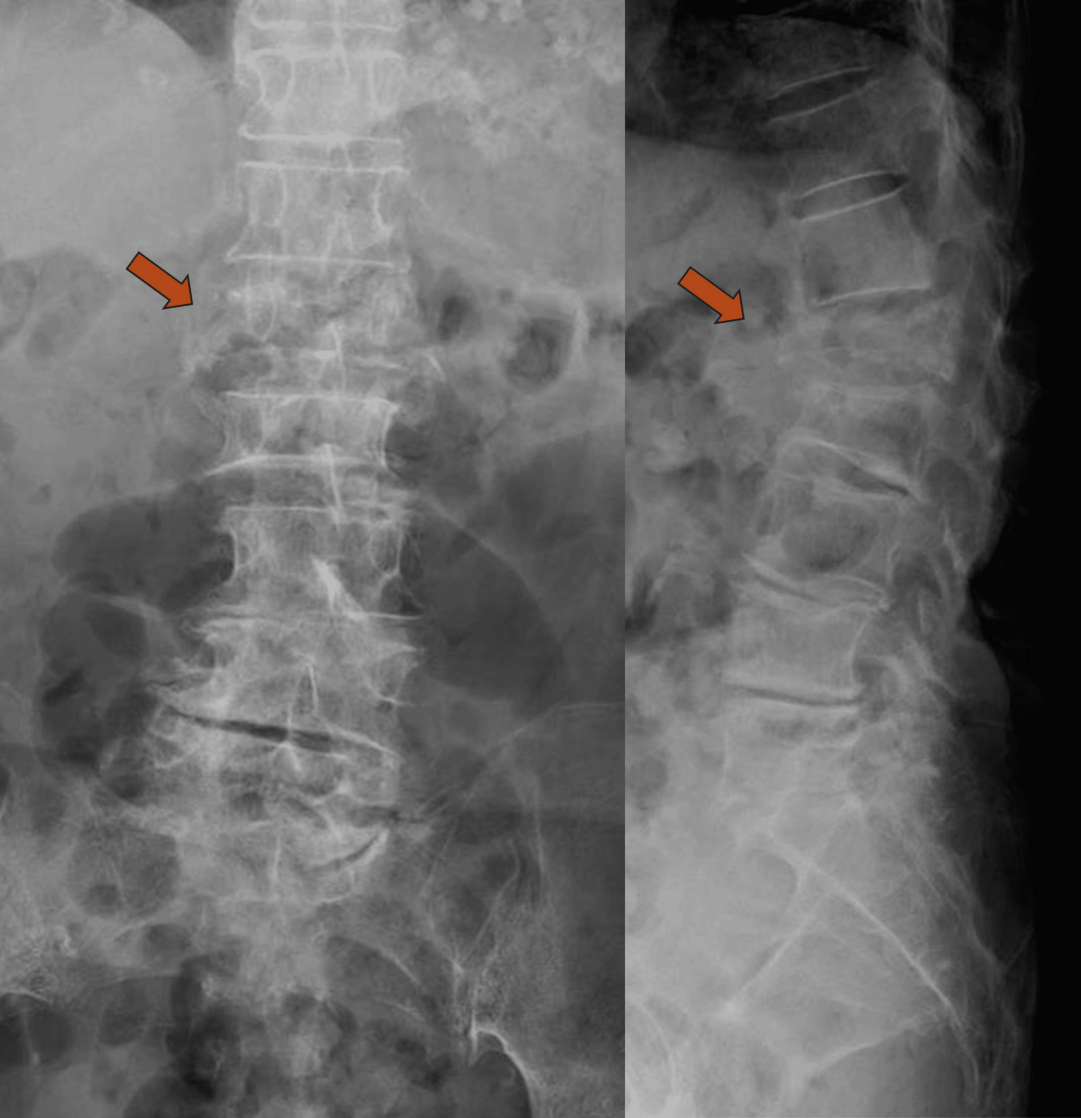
Preoperative X-ray showing an L1 compression fracture (arrow).
